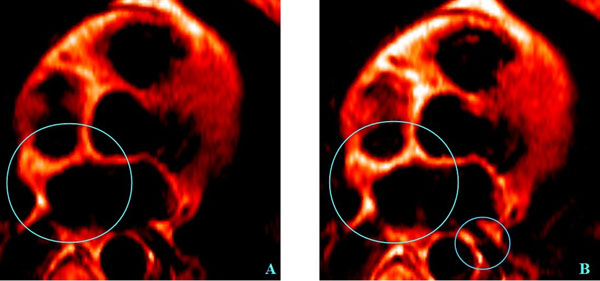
T1-weighted spin-echo CE MRI of the heart I a patient with a recent myocardial infarction and currently frequent SVT paroxysms. A - T1-w. SE MRI of the heart, with the axial slice position over the atrium, acquired before injection of paramagnetic contrast. B - T1-w. SE MRI of the heart in the same case, with the same position of the axial slice over the atrium, acquired injection of 15 ml of 0,5M paramagnetic contrast (as 2 ml per 10 kg of BM). The big circle depicts pathologic uptake of contrast to the atrial septum, ostium of the right pulmonary vein and interventricular septum, the little circle depicts the pathologic uptake of contrast to the ostium of the left pulmonary vein.
